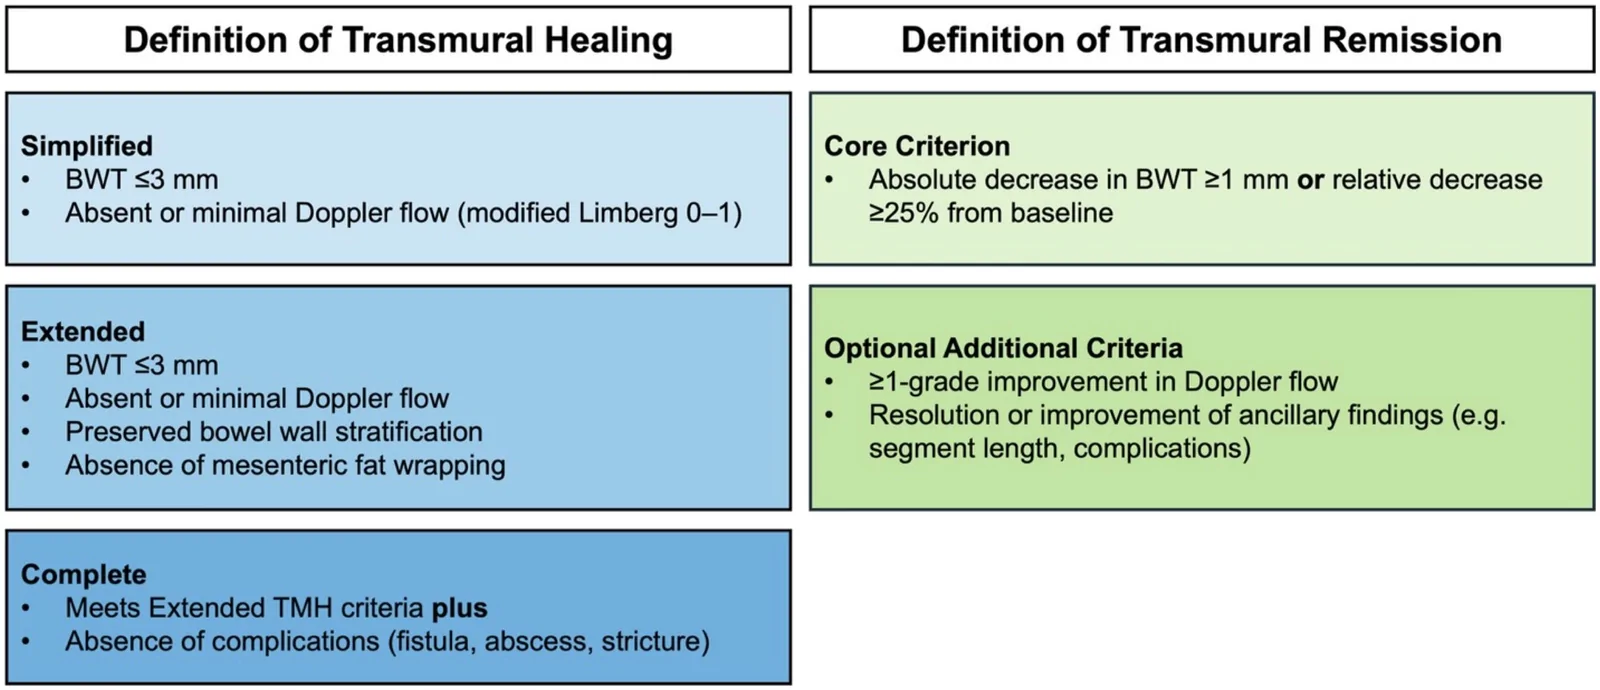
Proposed definitions of TMH and TMR on IUS in CD. TMH definitions are presented as a hierarchical framework with 3 tiers of increasing stringency: Simplified (BWT ≤ 3 mm and absent or minimal Doppler flow), extended (adds preserved bowel wall stratification and absence of mesenteric fat wrapping), and complete (adds absence of complications such as fistula, abscess, or stricture). TMR is defined by an absolute reduction in BWT ≥ 1 mm or relative decrease ≥ 25% from baseline, with optional criteria including Doppler improvement and resolution of ancillary findings.
